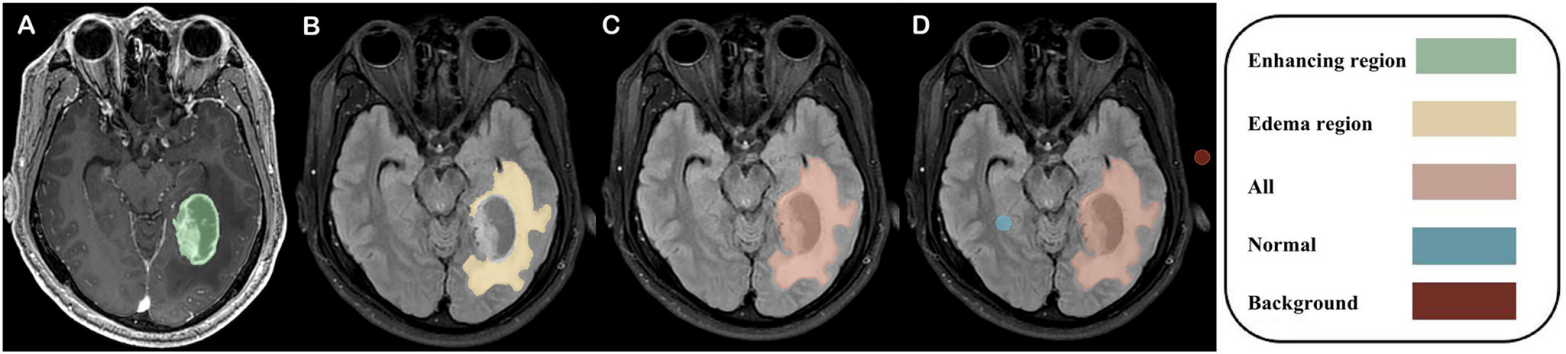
Representative case of *pTERTw* GBM. A 32‐year‐old male patient presented with a glioblastoma in the right temporal lobe. (A) The FLAIR_SD_ in the enhancement region was 47.39. (B) In the edema region, FLAIR_SD_ and rFLAIR were 69.29 and 1.54, respectively. (C, D) The FLAIR signal intensities of the whole lesion, contralateral normal region, and background were 867.37, 621.89, and 11.19, respectively. Nomogram analysis yielded a total score of 26, corresponding to a predicted probability of *pTERT* mutation of 0.06.
